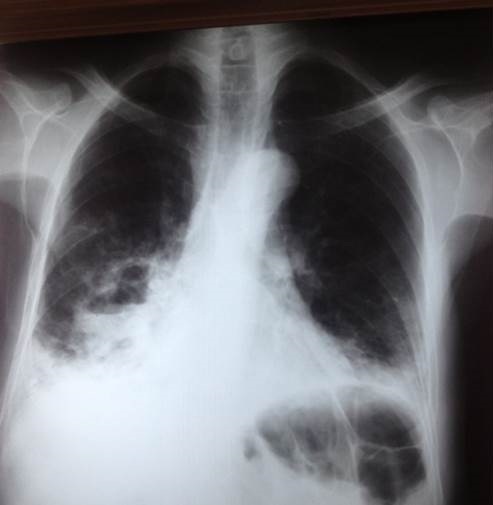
Radiographie thoracique de face objectivant deux opacités de type alvéolaires basithoraciques bilatérales excavés
